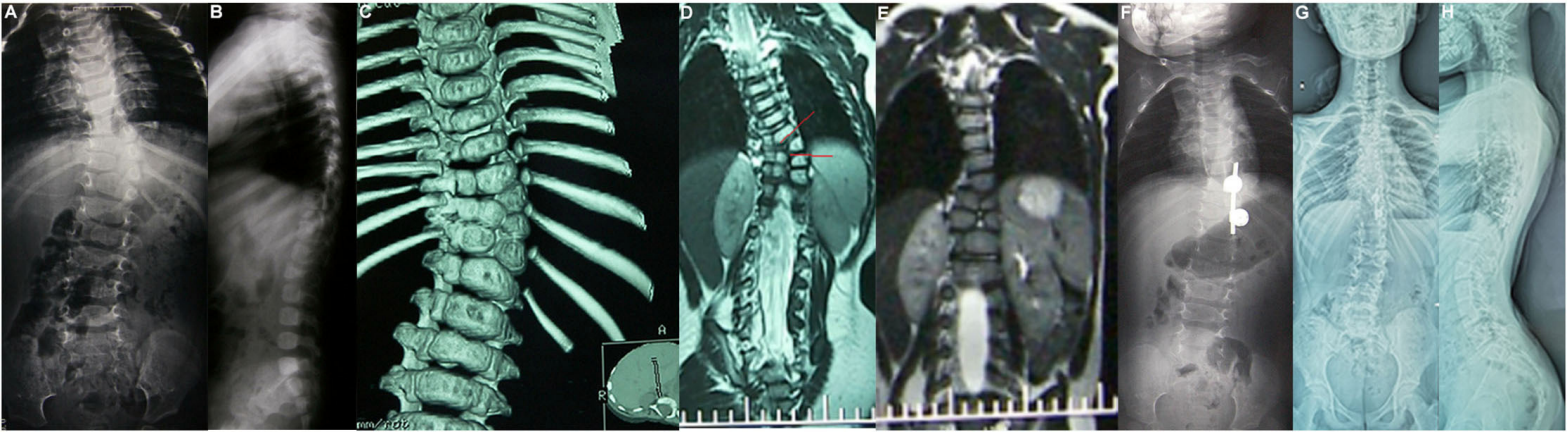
Female aged 1 years 4 months, T9–T10 hemivertebra; T8-12 bone bridge complicated with type II SCM, one-stage posterior T9–10 hemivertebra Schwab grade 5 osteotomy, T9-10 nail hook internal fixation, preoperative x-ray (A,B), preoperative CT (C,D), postoperative CT (E), postoperative x-ray (F) 1 year after operation, and the X-ray display was further improved 12 years after the last follow-up (G,H).
